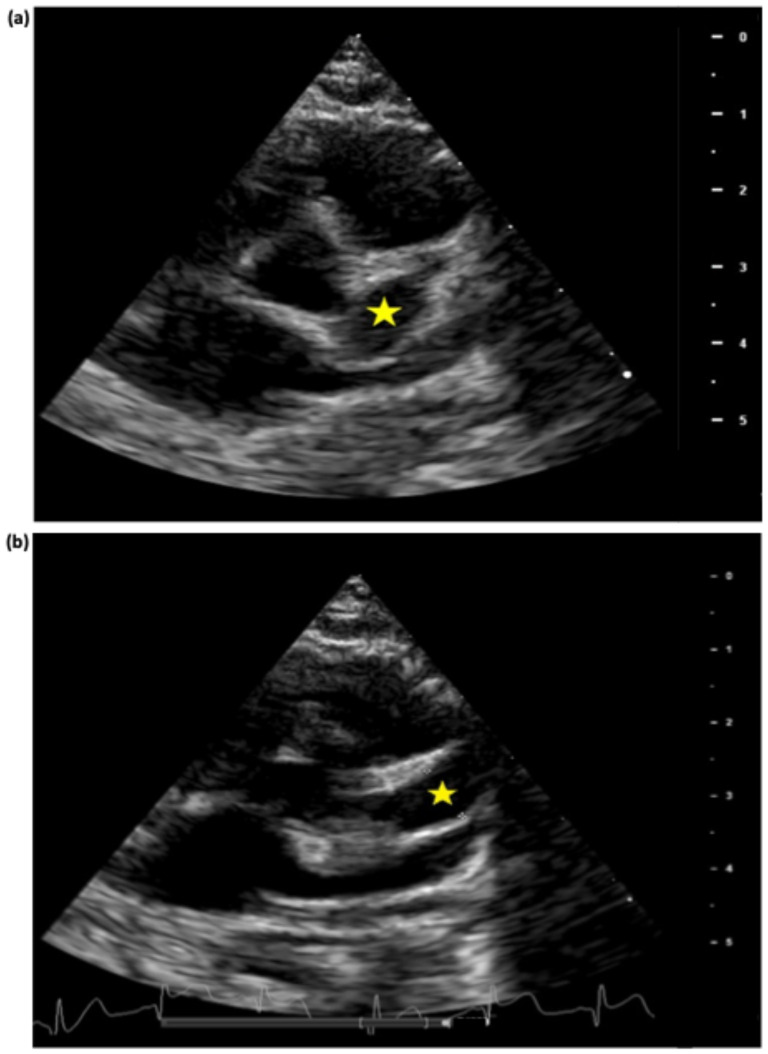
Echocardiogram performed on day 57 of illness, parasternal short axis view, showing left anterior descending artery proximal aneurysm with diameter of 5.5mm, z-score +18 (a), and left anterior descending artery distal aneurysm with diameter of 7.5mm, z-score +27 (b). The stars indicate the aneurysms. Scale in centimeters on the right-hand side of each image.
